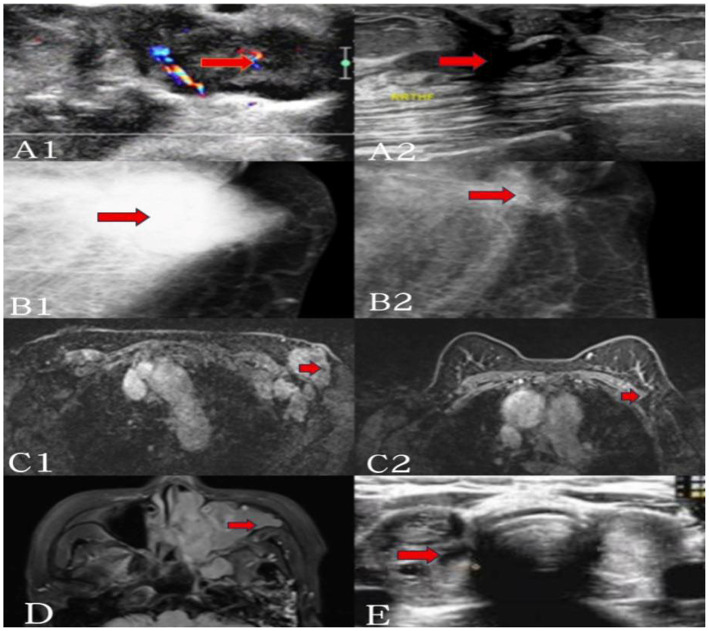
(A1,A2) Contrasting breast color Doppler ultrasound images of the left axillary mass before and after neoadjuvant therapy. (B1,B2) Contrasting mammography images of the left axillary mass before and after neoadjuvant therapy. (C1,C2) Contrasting magnetic resonance imaging of the left axillary mass before and after neoadjuvant therapy. (D) A nodular abnormal signal focus is observed in the left nasal cavity. It exhibits isointense signal on T1-weighted imaging (T1WI), slightly hyperintense to isointense mixed signal on T2-weighted imaging (T2WI), and slightly hyperintense signal on FLAIR. The margins are relatively well-defined, with dimensions approximately 61 mm × 24 mm × 19 mm. The lesion extends posteriorly into the nasopharyngeal cavity. (E) Pre-treatment color Doppler ultrasound of thyroid mass.
